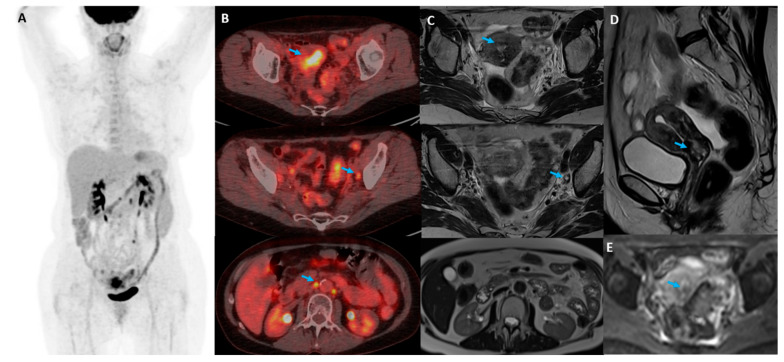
Posttreatment discordant early 18FDG PET/CT and MRI results with 6-month follow-up. Maximal intensity projection (MIP) 18F-FDG PET/CT reconstruction (A) indicates the presence of persistent metabolically active cervical cancer and pelvic and paraaortic lymph nodes, visible on fused PET/CT axial slices (column (B)) on three different levels (blue arrows). Residual cancer presence is indicated (blue arrows) on the same levels on T2w axial pelvic images (column (C)), T2w image in sagittal plane (D), and DWI (b = 800 s/mm^2^) image in axial plane (E). MRI failed to detect the presence of paraaortic lymph node metastasis.
